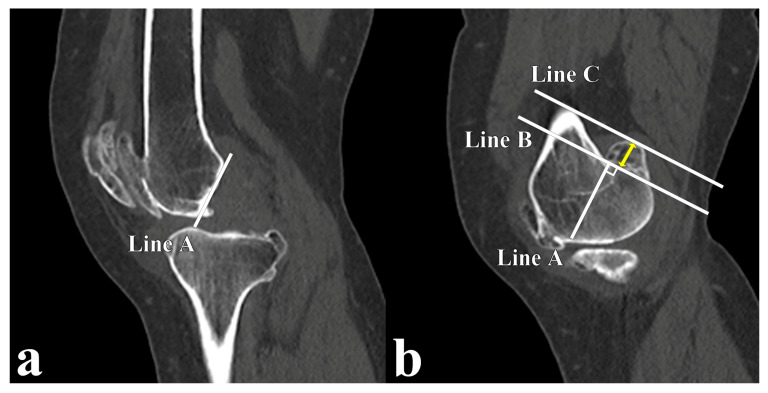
Technique of osteophyte size measurement. (a) Midsagittal section of CT: A line is drawn on the Blumensaat line extending from the anterior to the posterior-most aspect of the femoral cortex (Line A). (b) Sagittal section of CT with largest osteophytes: Line A is copied to sagittal section of CT with largest osteophytes, and second line (Line B) is drawn on the most posterior aspect of femoral cortex, perpendicular to Line A. A third line (Line C) is drawn parallel to line B and on the most posterior edge of osteophyte. The distance between Line B and Line C (yellow double-headed arrow) is recorded as the osteophyte size. CT: computed tomography.
